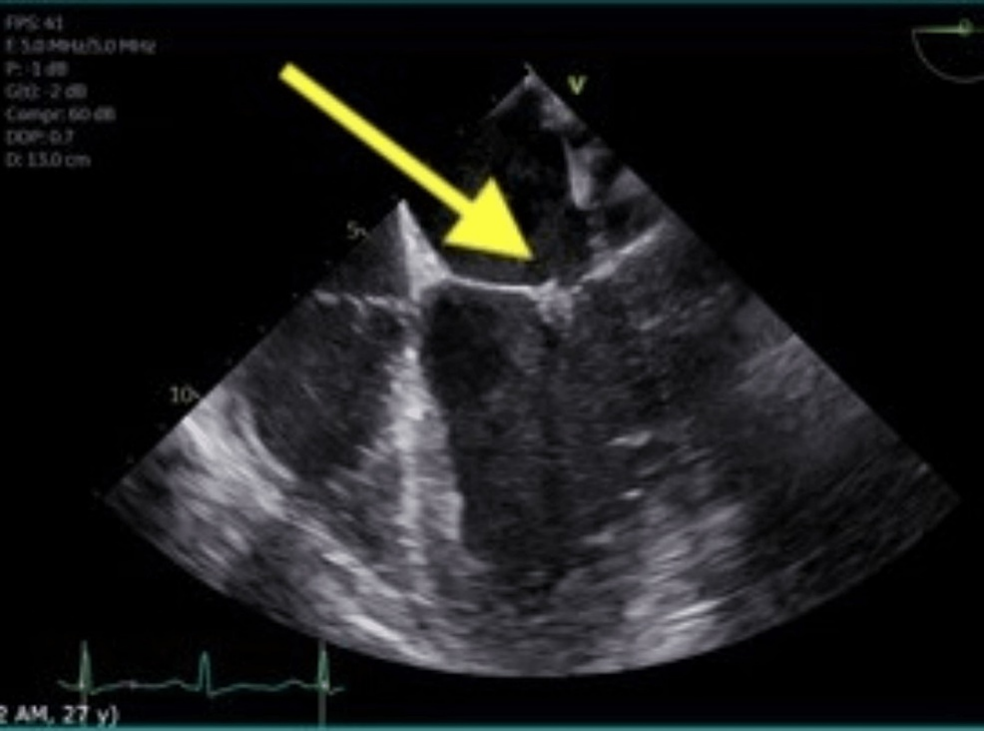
Transesophageal echocardiogram showing sessile densities (yellow arrow) of the mitral valve leaflet tips at the co-aptation points
